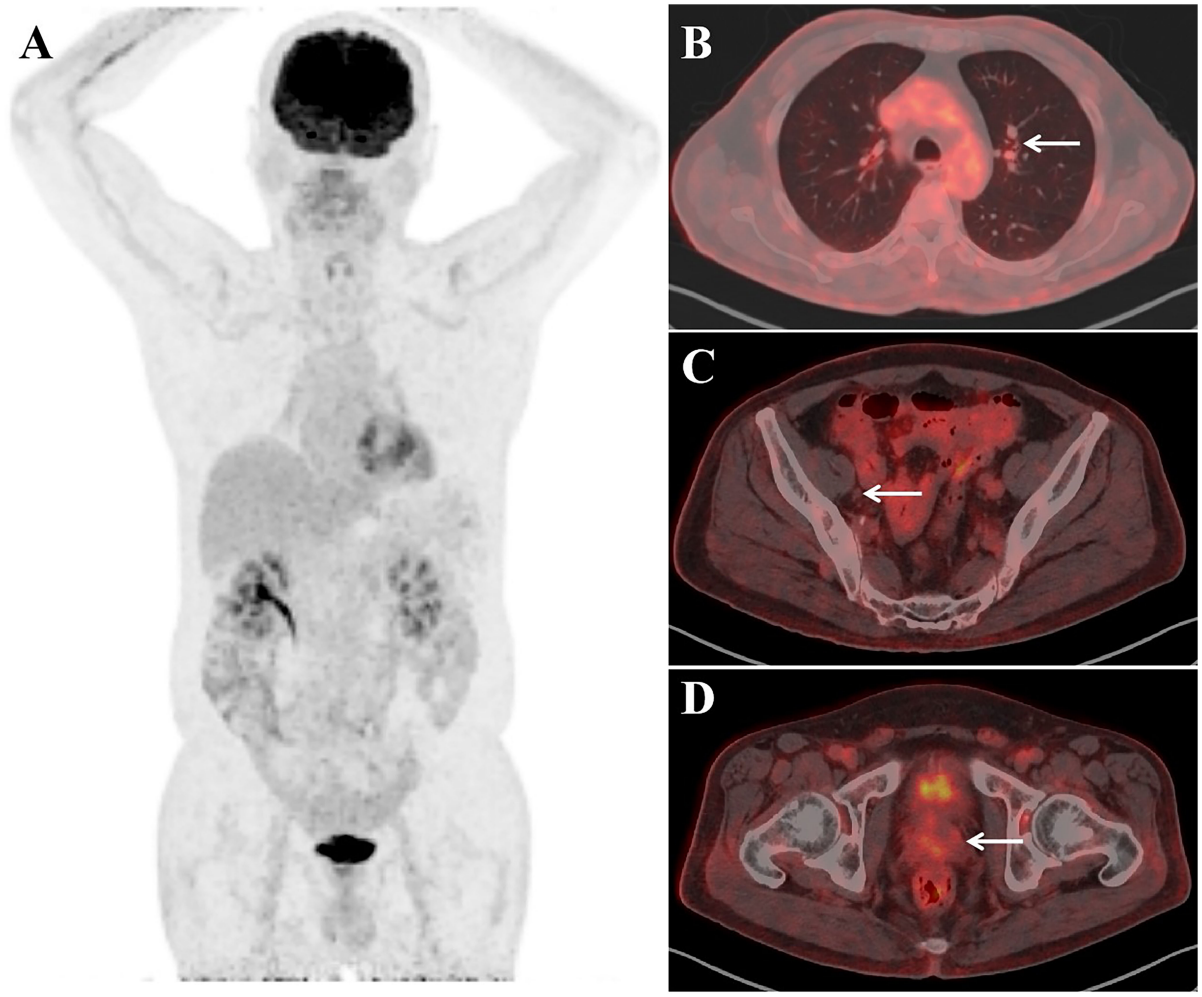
Imaging studies after the treatment of ADT combination with PARPi for two months. PET maximum-intensity-projection image (MIP) (A), Fused axial (B–D) images showed no evidence of FDG–concentrating active disease foci.
